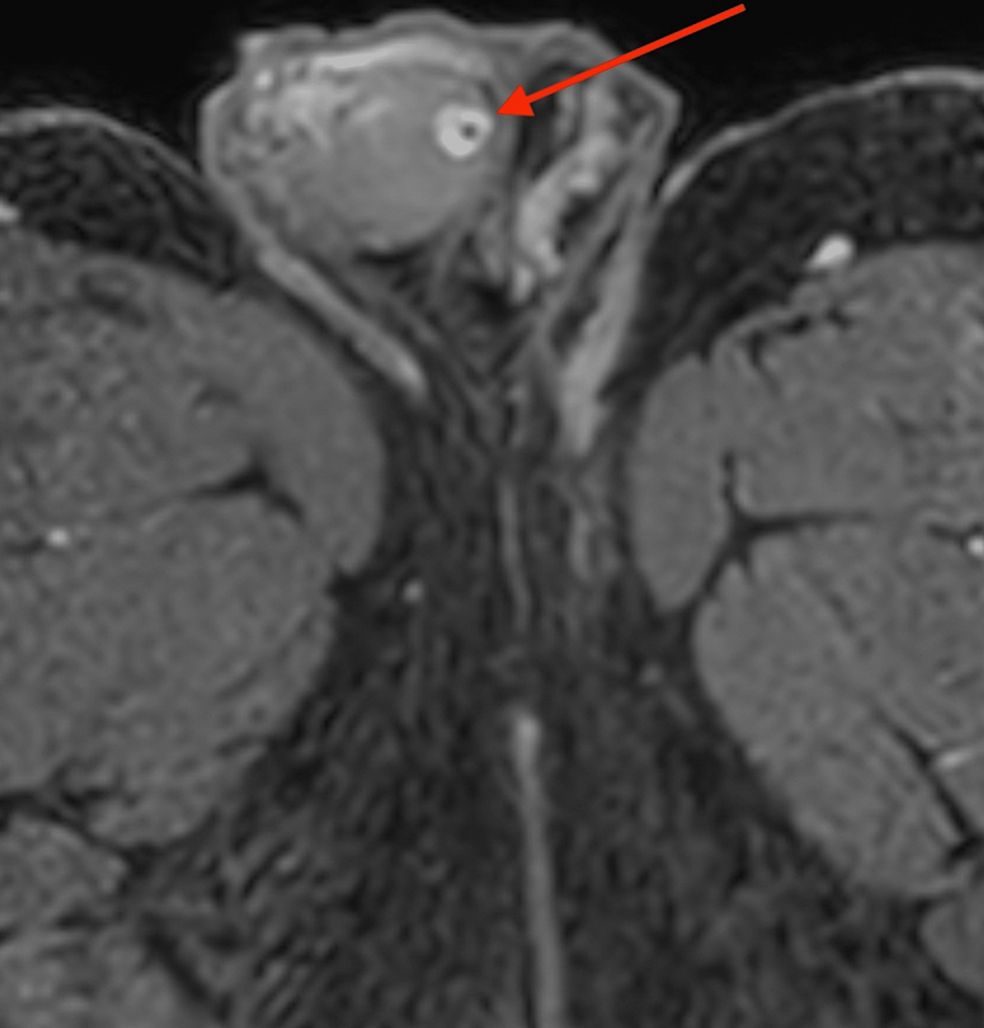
Contrast-enhanced, fat-suppressed T1-weighted transversal MRI of the pelvis Image of the scrotum and right testicle and transversal view of both thighs showing a ring-shaped, strong enhancement with contrast medium sparing of the hypo-intense tumor center in the right testicle (marked with the arrow)
